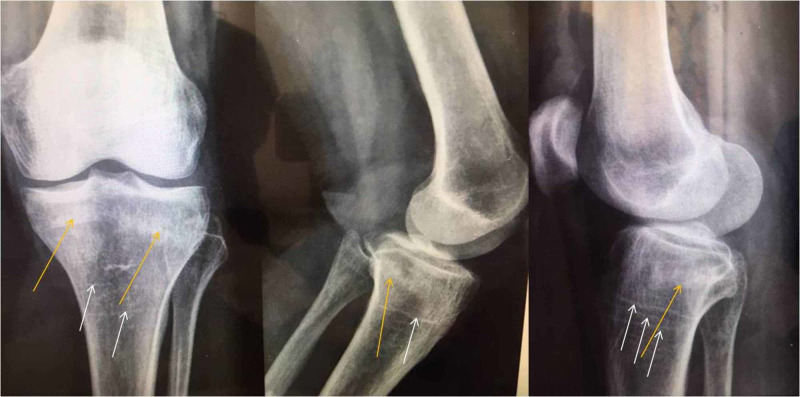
X-rays with callus formation (yellow arrows) implying fracture healing. Also, note the growth arrest or Harris lines (white arrows) that usually result from pathologic levels of stress during bone development.
